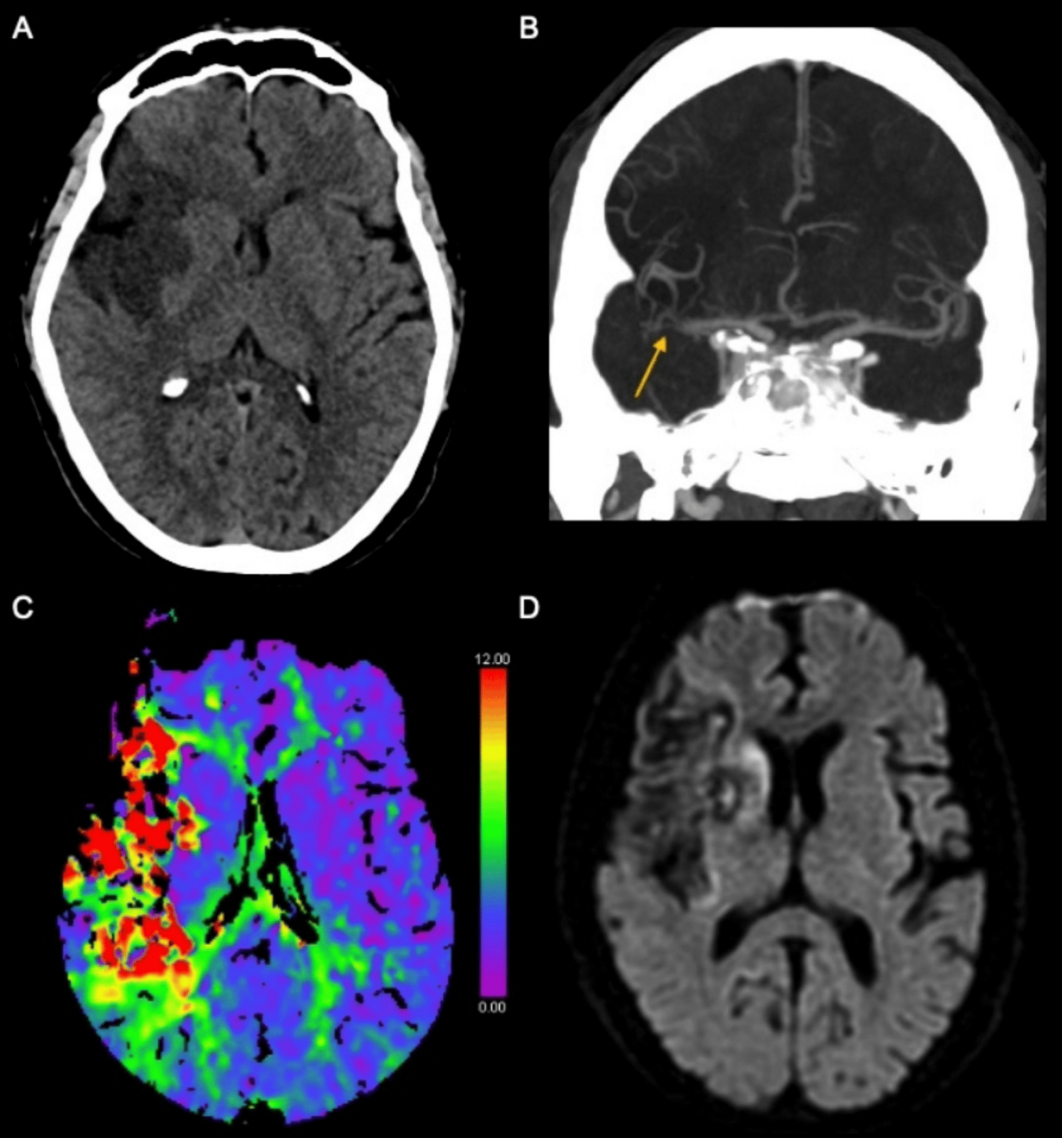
(A) Noncontrast CT shows a large hypodense infarct in the right MCA territory. (B) Coronal CT angiography demonstrates reduced caliber of the distal right M1 segment (arrow). (C) Color-coded Tmax map shows delayed perfusion within the right MCA territory without hypoperfusion in the contralateral hemisphere. (D) Diffusion-weighted imaging obtained one month later shows subacute infarction in the right MCA territory, with no diffusion abnormalities in the left hemisphere MCA: middle cerebral artery
